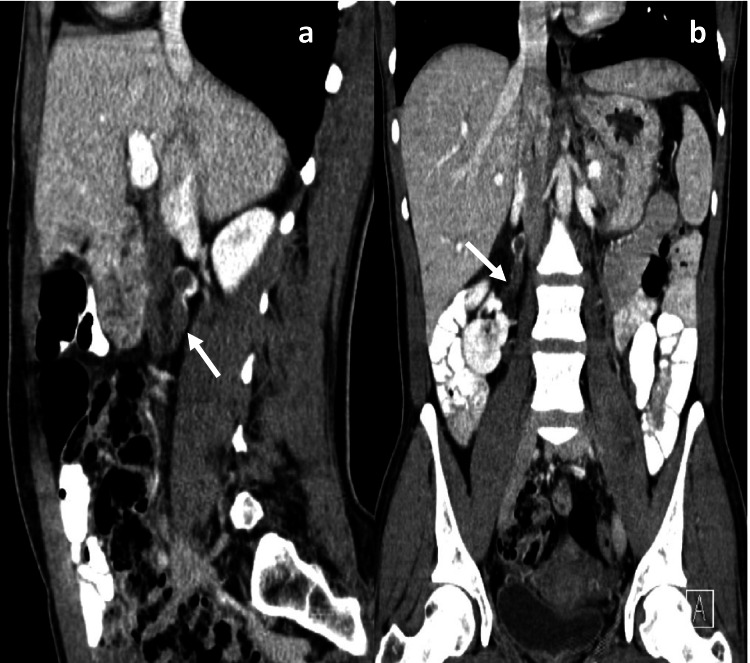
Post-contrast CT images Sagittal (a) and coronal reformatted (b) post-contrast CT images of the same patient demonstrate the superior extent of the thrombus into the upper part of the right ovarian vein (white arrows). This patient was treated by the authors at King Abdulaziz University Hospital.
